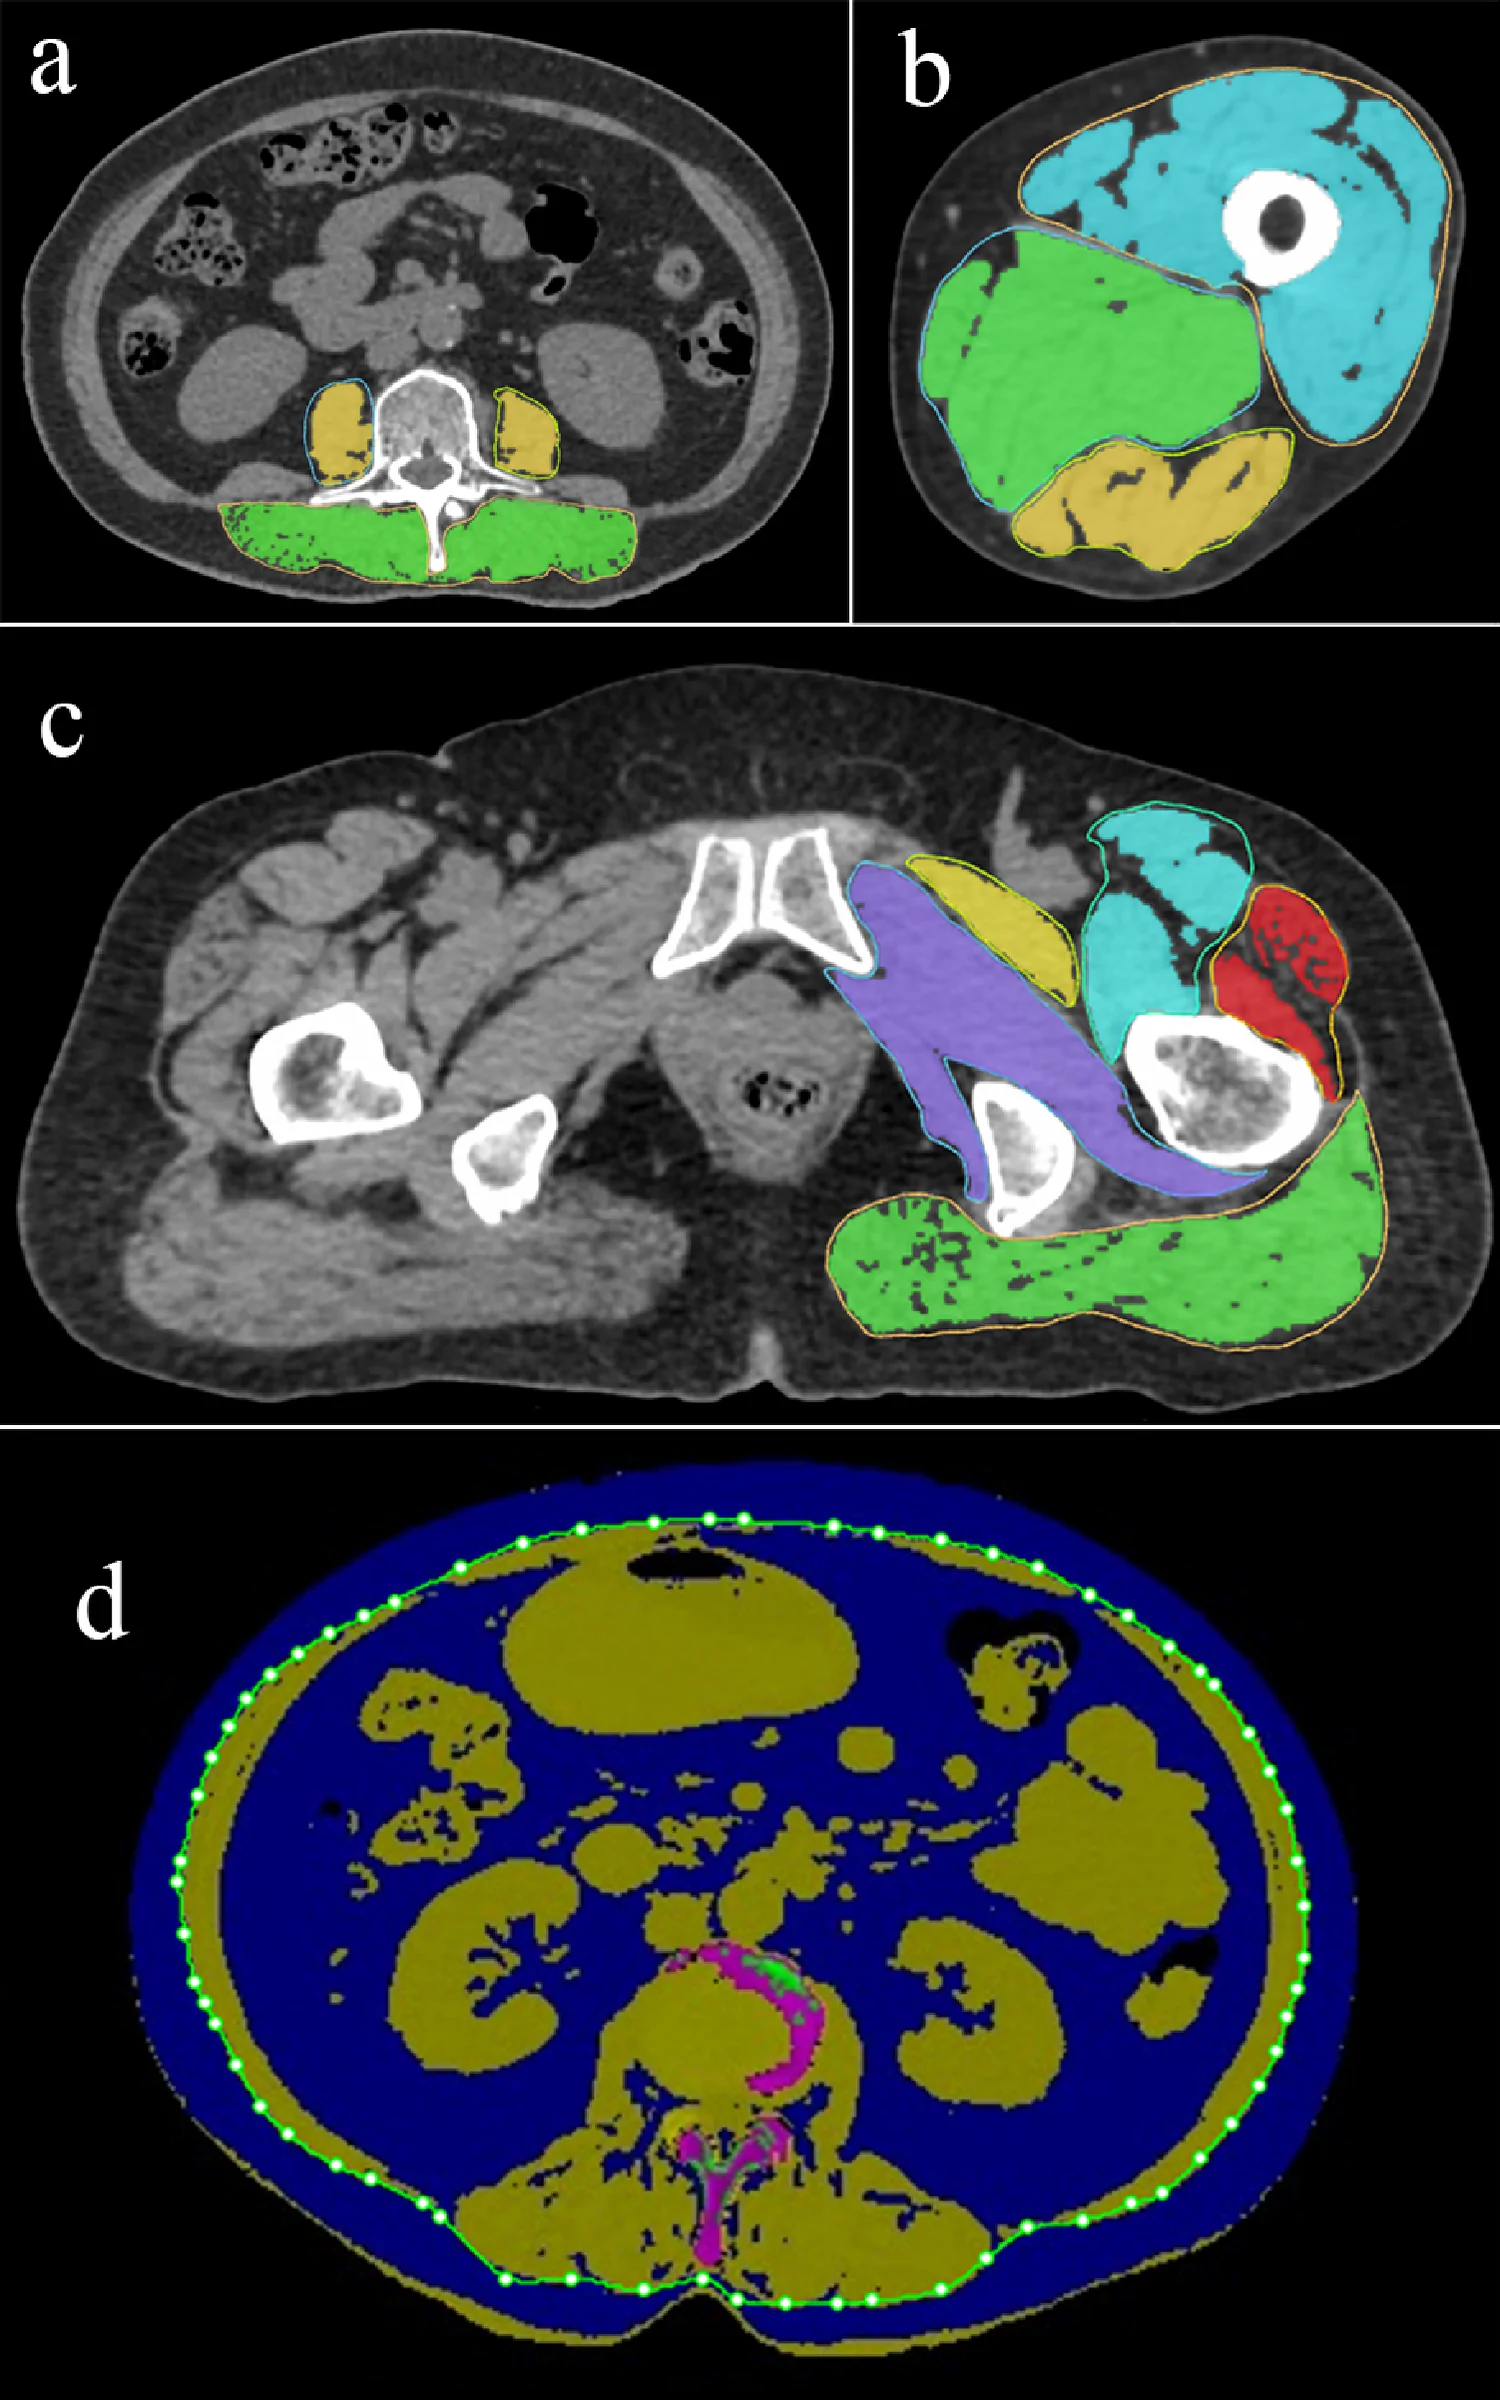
(a) Measurement of cross-sectional area and mean computed tomography (CT) values of the paraspinal (green) and psoas major (yellow) at the level of L3; (b) Measurement of the anterior compartment (blue), the medial compartment (green) and the posterior compartment (yellow) of thigh muscles at the level of mid-thigh; (c) Measurement of extensors (green), external rotators (purple), adductors (yellow), flexors (blue) and abductors (red) at the level of the lesser trochanter; (d) Measurements of total adipose area (TAA) and visceral adipose area (VAA) were semi-automatically completed, and green dots show the region of interest demarcating visceral and subcutaneous adipose tissue at the L3/4 level
